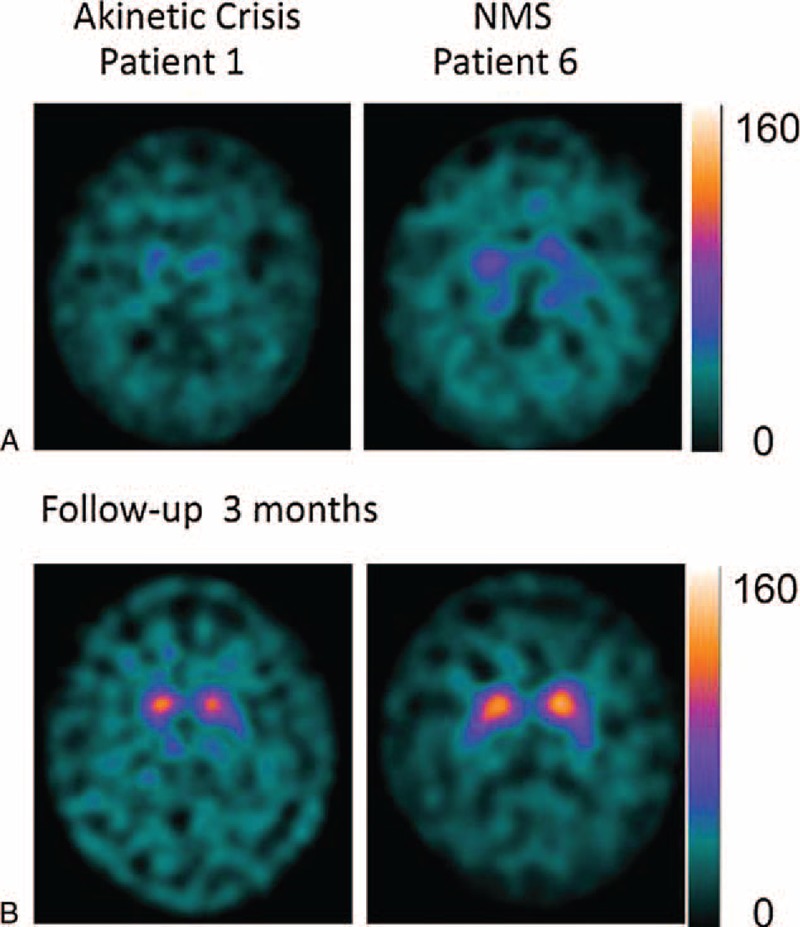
FP/CIT SPECT acquisition during AC/NMS, and after recovery, in patient 1 and 6. Notice almost complete disappearance of binding during the crises (“burst striatum”) and recovery (A), 3 months after the crises (B), with appearance of an “egg shape” pattern in patient 1 and a “mixed type” pattern in patient 6. Notice that saturation level scales are 4 times higher than the scales reported in figure 1. AC = akinetic crisis, NMS = neuroleptic malignant syndrome, SPECT = single-photon emission computerized tomography.
